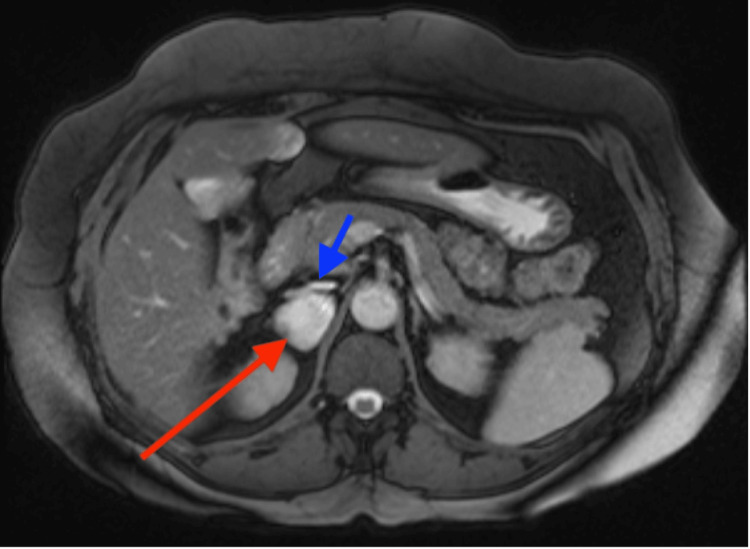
Axial T2 MRI demonstrating the IVC (blue arrow) being compressed by the RBC (red arrow) IVC: inferior vena cava; MRI: magnetic resonance imaging; RBC: retroperitoneal bronchogenic cyst
